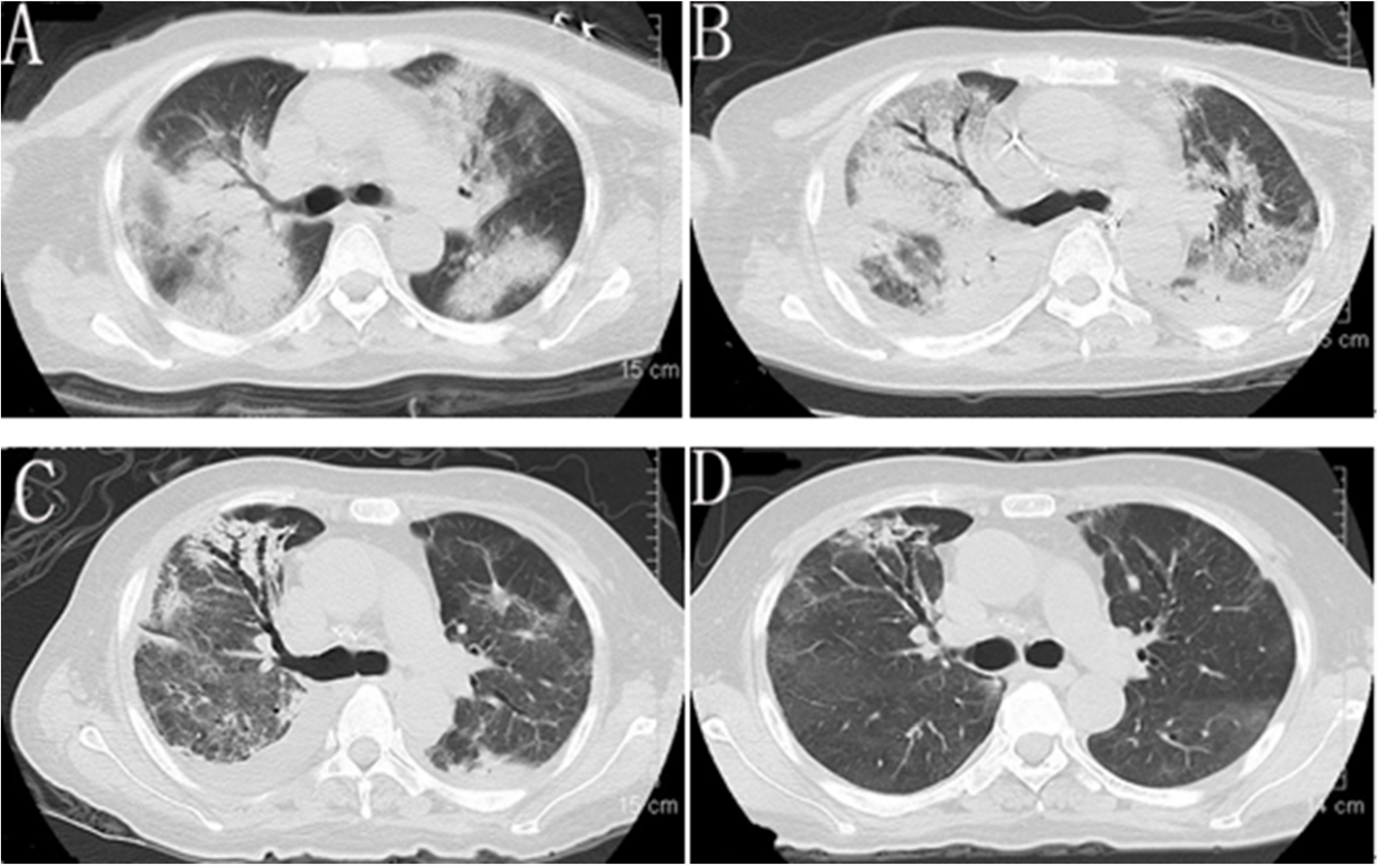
Chest computed tomography scan at admission (A), 14d later (B), 22d later (C) and 47d later (D)
